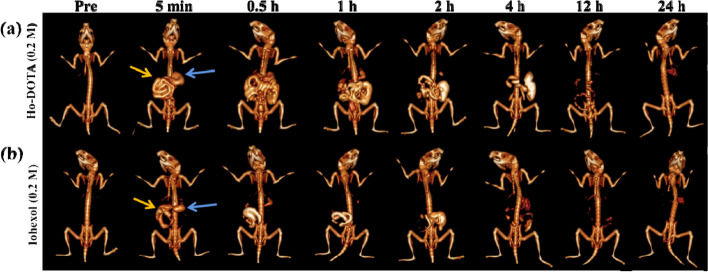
In vivo GI tract CT imaging after oral administration of (a) 0.2 M Ho-DOTA, (b) 0.2 M iohexol (blue arrows represent stomach and yellow arrows represent small intestine)
